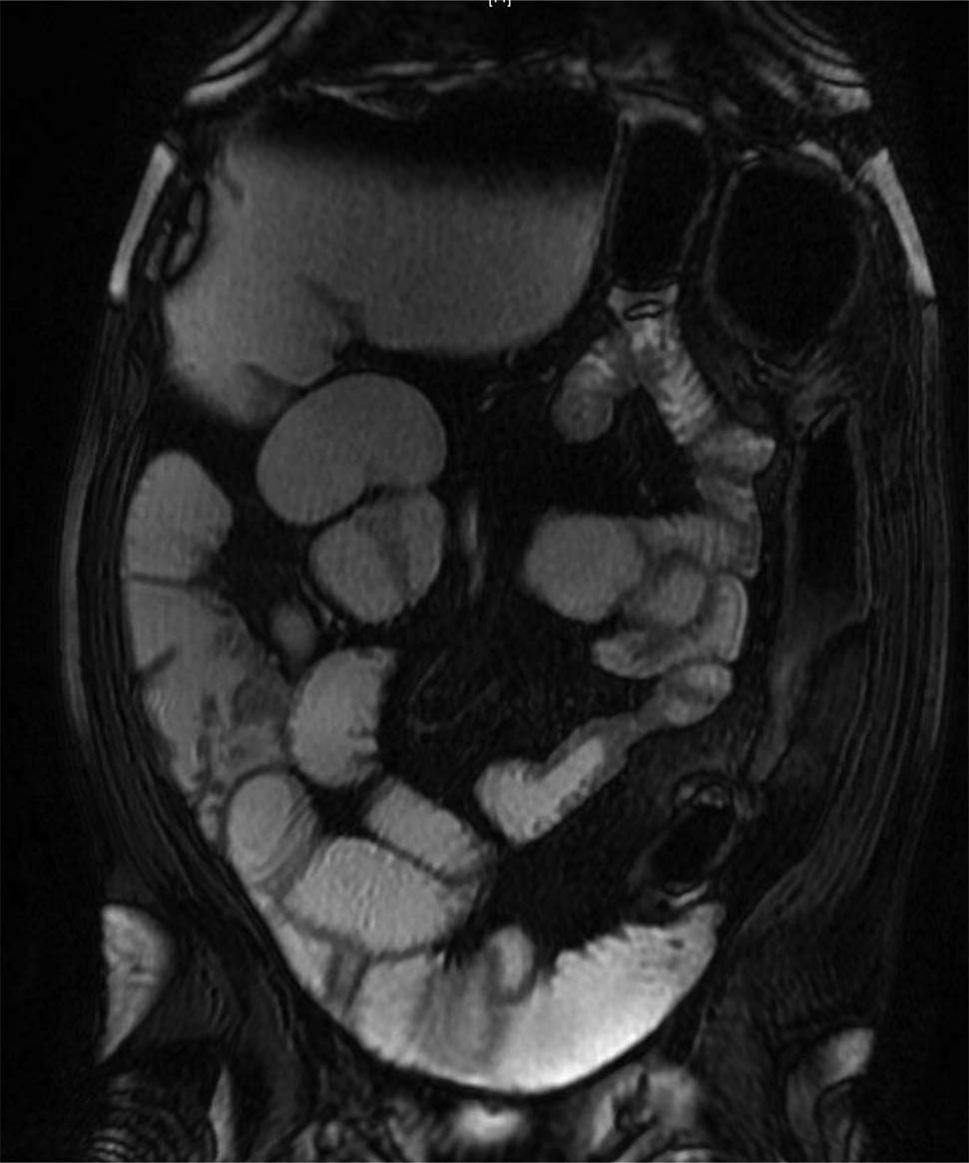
Magnetic resonance image showing a 4 cm long colon's fibrotic stenosis.
